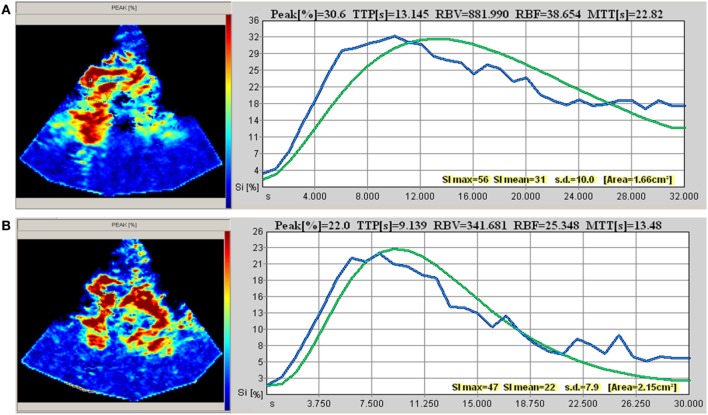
Transcranial perfusion sonography (example taken from patient 2). (A) Baseline measurement [before optimization of cardiac index (CI)] at CI 2.1 and mean arterial pressure (MAP) 89 mmHg. (B) Second measurement (after optimization of CI) at CI 3.45 and MAP 89 mmHg.
